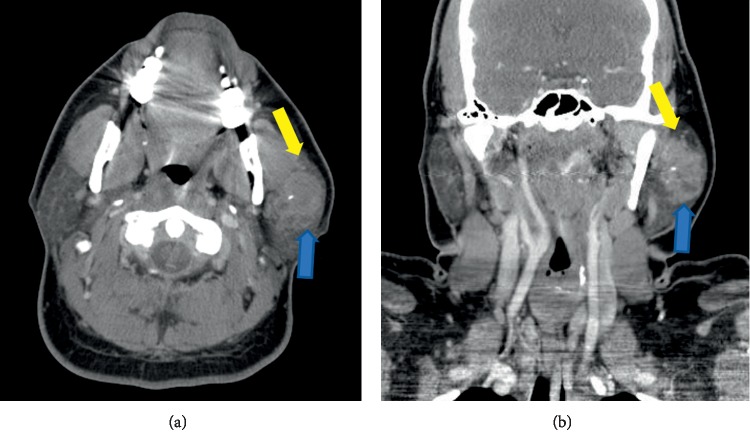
Axial (a) and coronal (b) contrast-enhanced CT images show a complex lobular mass in the left parotid gland with heterogeneous enhancement including hypodense areas of necrosis (blue arrows) and a central hyperdensity indicating a calcified component (yellow arrows).
